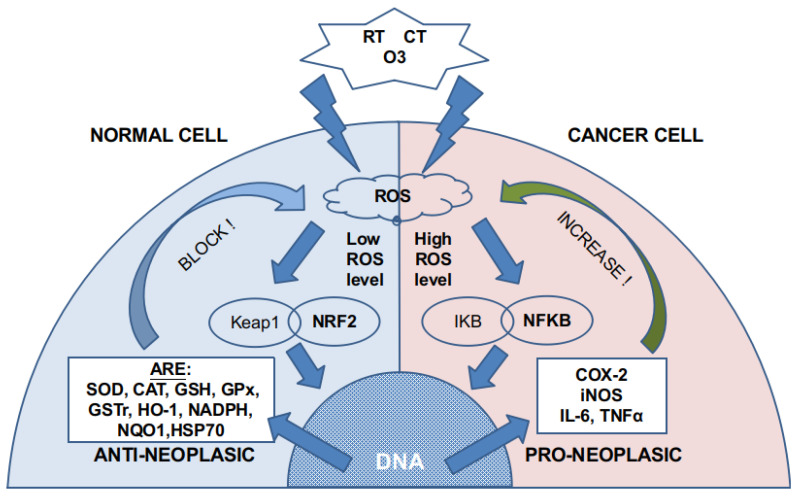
ROS generation by RT, CT, and ozone. According to previous cytoplasmic and mitochondrial ROS levels, different pathways are activated. Normal cells have a low ROS level, whereas cancer cells have high or very high ROS levels. The NRF2 pathway is almost exhausted in cancer cells, so more ROS activate the NFKB pathway.
